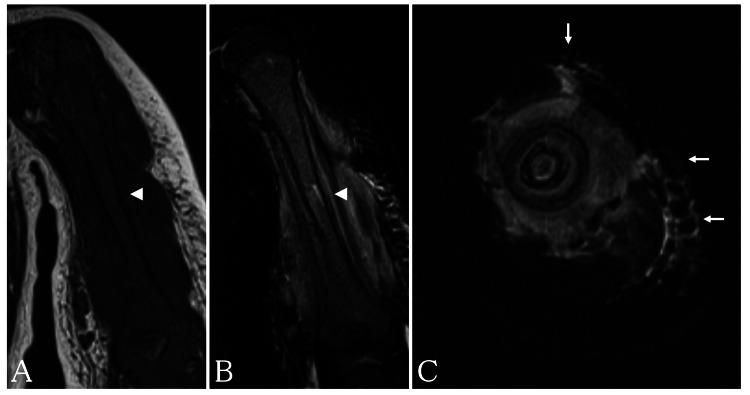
Magnetic resonance image of the left humerus. Coronal T1-weighted image shows diffuse low signal intensity in the left humeral bone marrow (arrowhead) (A), and a coronal short tau inversion recovery (STIR) image depicts local high signal intensity in the left humeral bone marrow (arrowhead) (B). Axial STIR image reveals high signal intensity in the subcutaneous tissues adjacent to the brachial muscles (arrows) (C).
